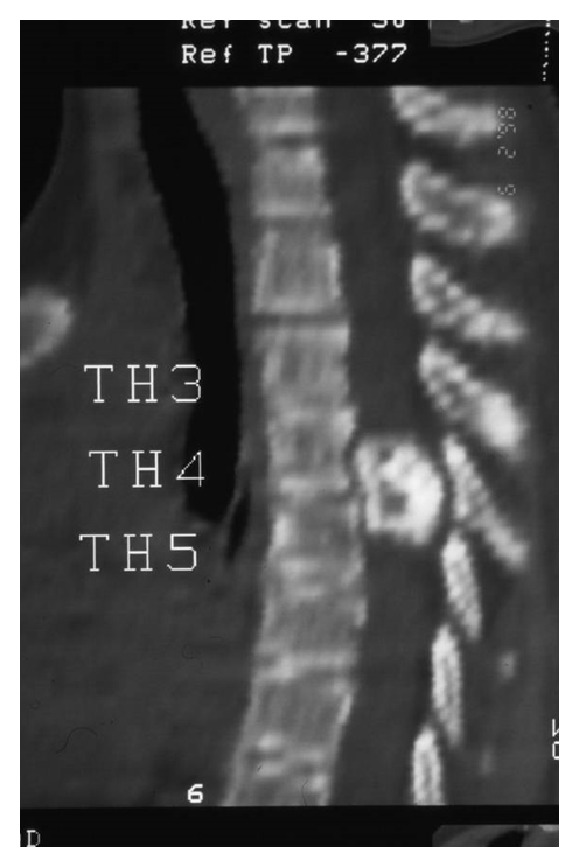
Reformatted sagittal CT scan showed a lesion arising from the T3–5 lamina and indenting the spinal cord (obliteration and stenosis of the spinal canal at the level of T3).
